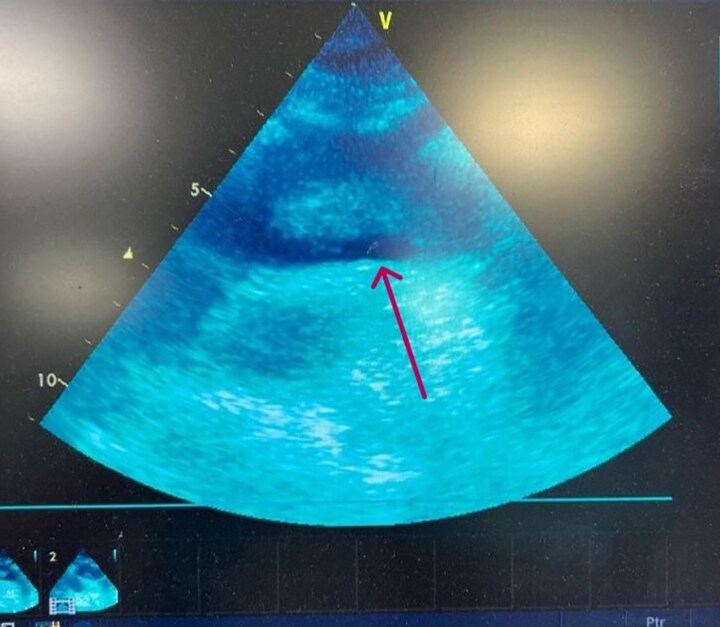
Transoesophageal echocardiography showing a free-floating thrombus within the descending thoracic aorta (indicated by arrow). The thrombus appears as an echogenic mass with a point of attachment to the aortic wall, consistent with findings observed on CTA.
